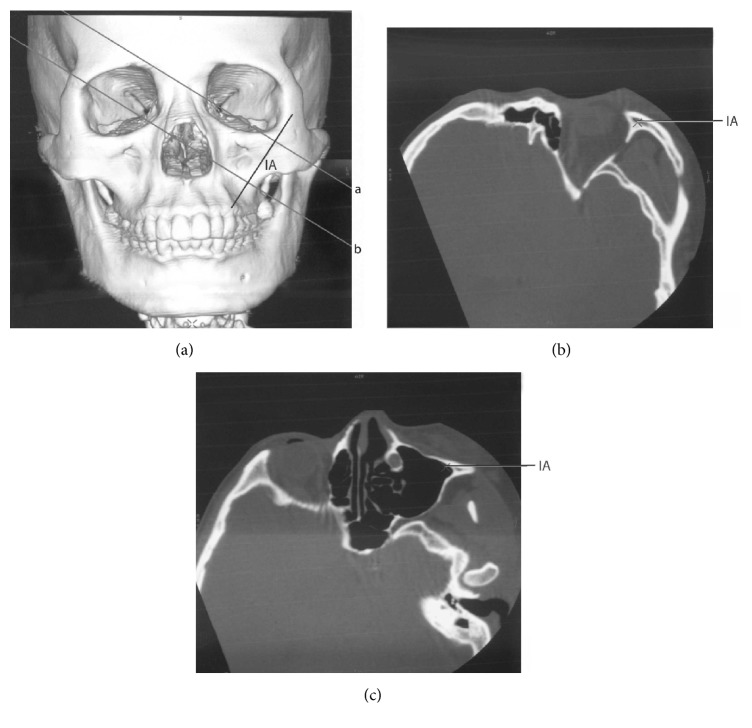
The implant axis (IA) and its surrounding structures. Lines a and b in (a) represented the two planes vertical to IA in the 3D-CT image in (a). Line a went through the bone body of zygoma, while line b went through the maxillary sinus. (a) 3D-CT image of the head. (b) The plane that line a went through, showing the structures around IA in the zygoma. The marked point in the figure indicated IA. (c) The plane that line b went through, showing the position of IA and the maxillary sinus. The marked point in the figure indicated IA.
